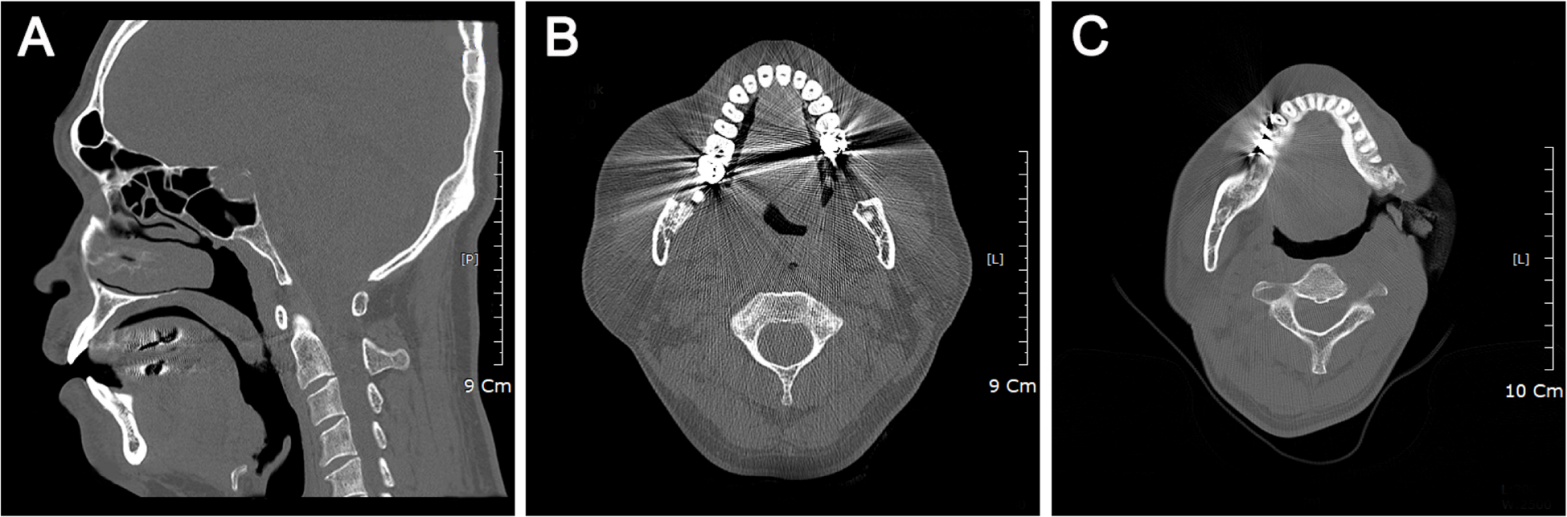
a Computed tomography reveals swollen oral mucosa enlargement of the soft palate and uvula in the sagittal plane. b Transverse plane. c Computed tomography shows bone defect in the left mandible angle and ramus and a skin defect in the left mandibular area with an orocutaneous fistula from complications of radiation therapy following surgery for gingival cancer
